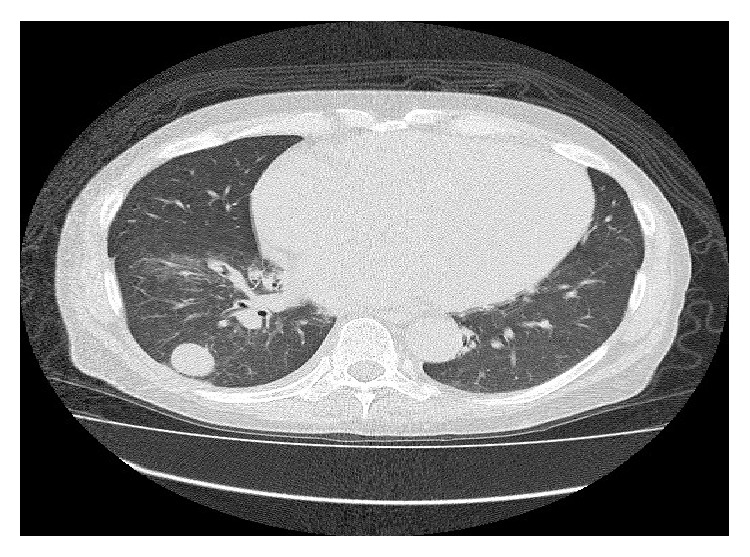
CT showing lung lesion before Vemurafenib.
